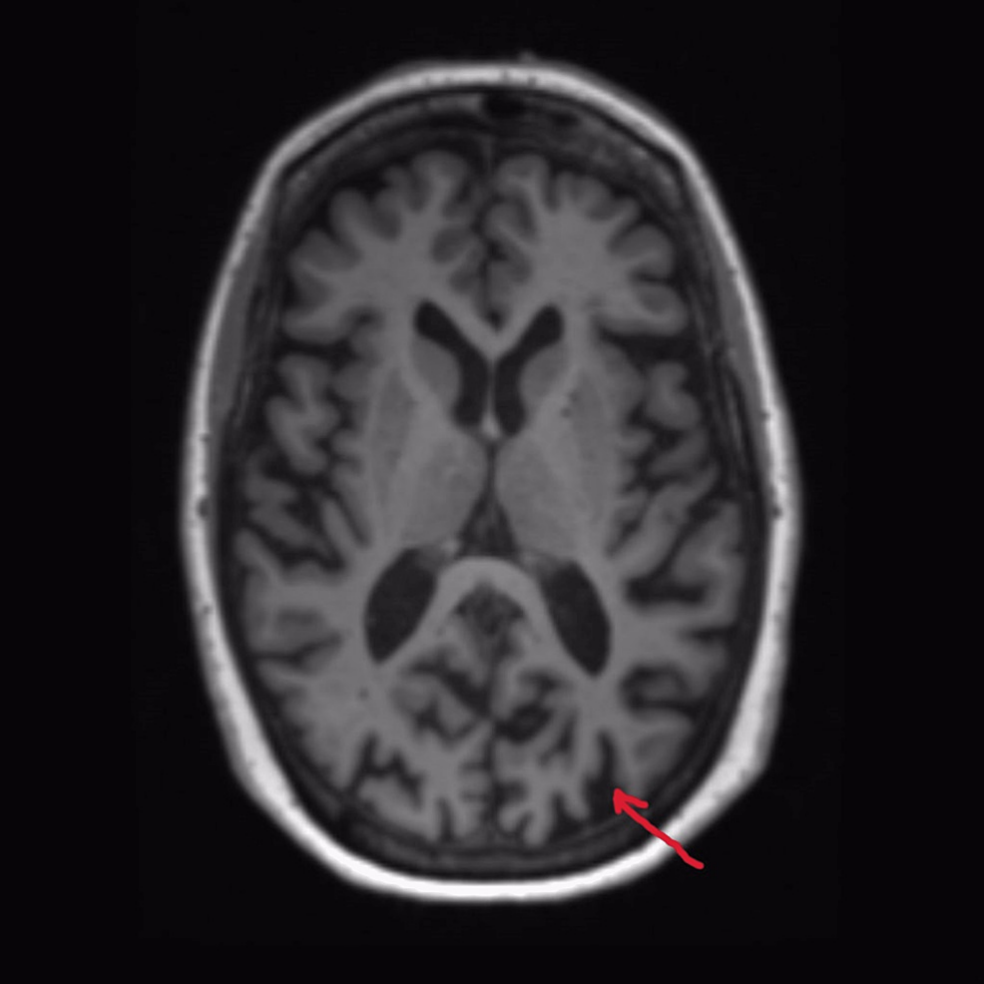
MRI Brain Axial T1 indicating generalised cortical atrophy with prominent atrophy in parietal and occipital regions
